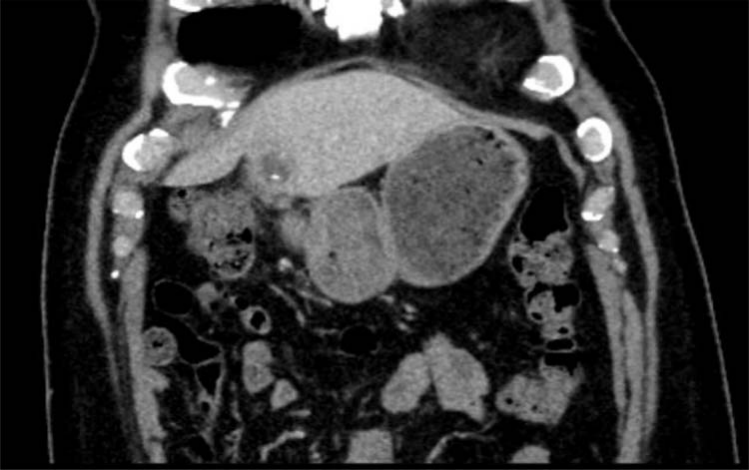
CT scan showing left lobe liver abscess with fishbone.
